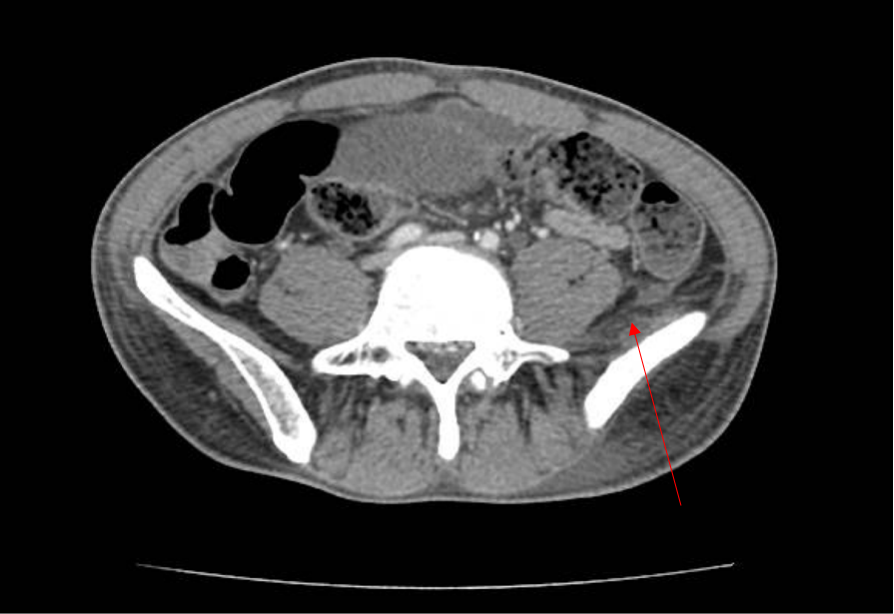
CT Abdomen and Pelvis cut demonstrating an irregular rim-enhancing fluid collection centred over the left medial thigh, involving the left abductor muscles, extending superiorly to involve the obturator externus and iliacus muscles to the level of the iliac crest
